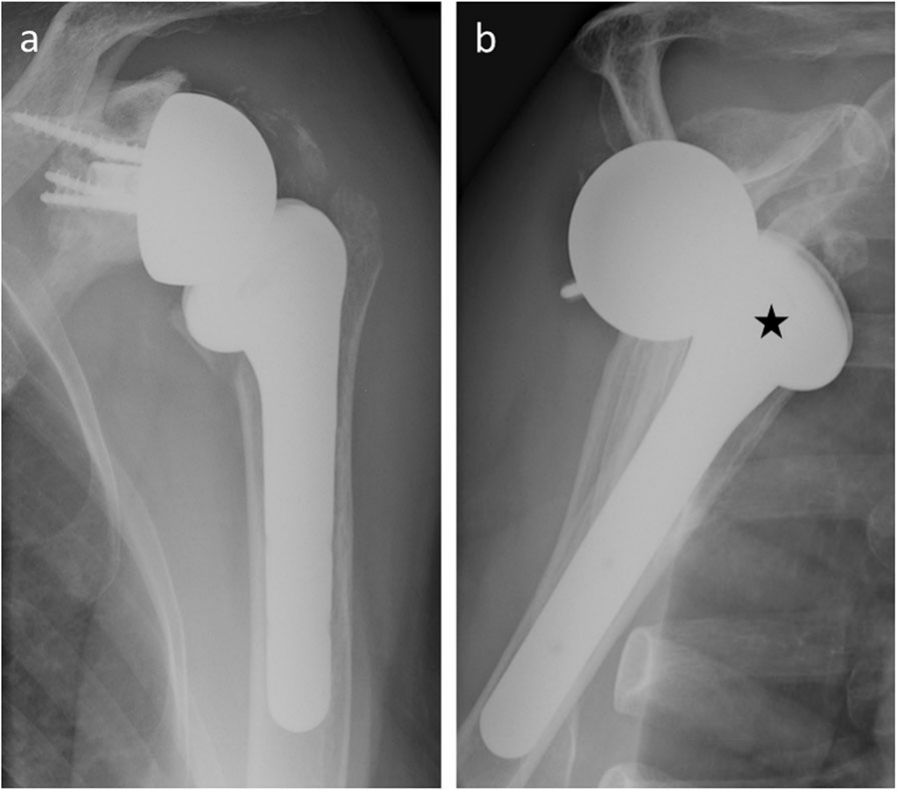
Anteroposterior (a) and scapular Y (b) radiographs of a 76-year-old woman with reverse shoulder arthroplasty (2 months after surgery) show an evident anterior dislocation (star) in scapular Y view. Note that this dislocation is difficult to highlight on anteroposterior view
